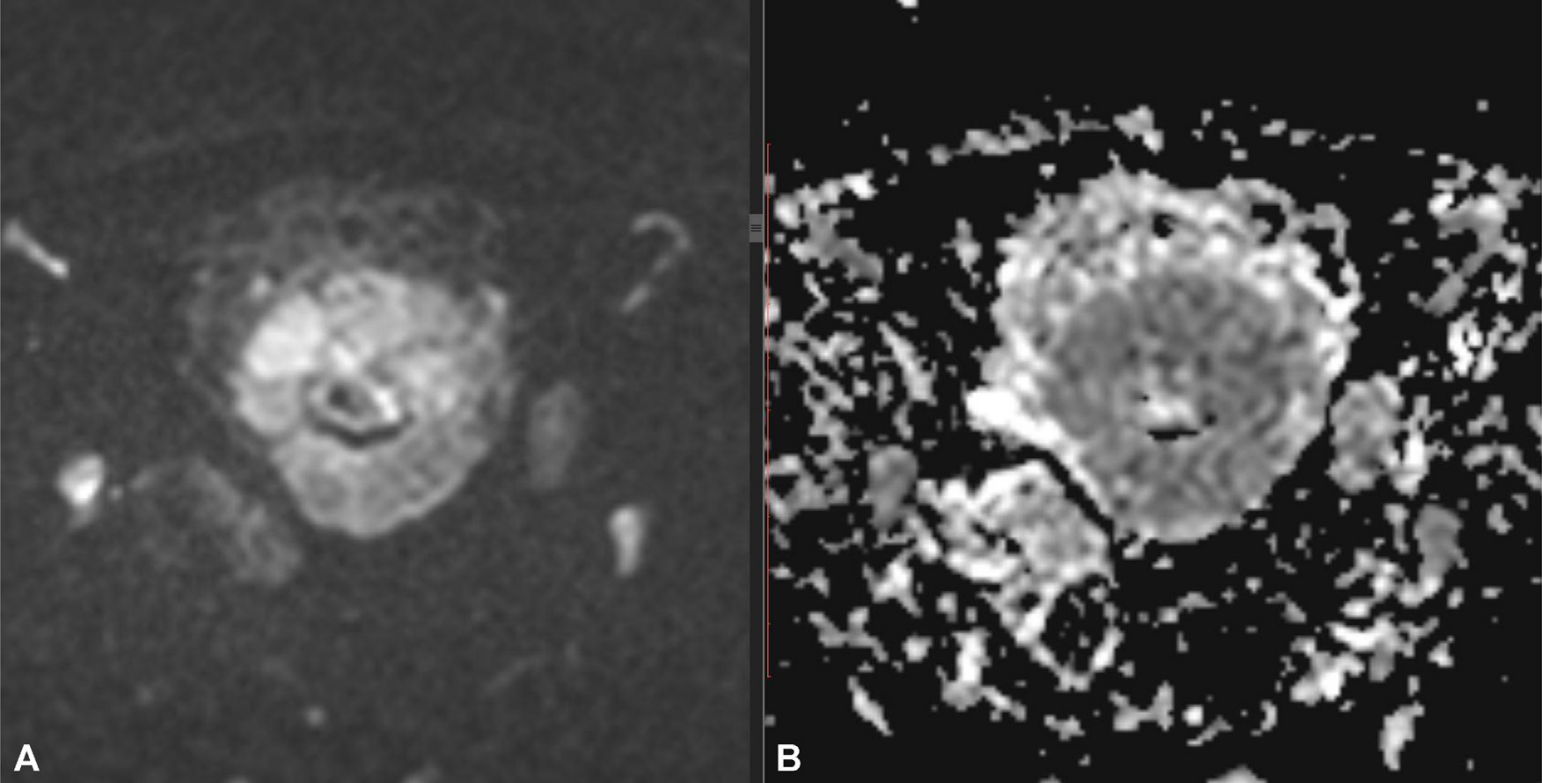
Diffusion weighted images. Infiltrative uterine mass show restricted diffusion seen as hyper intense signals on DWI and hypo intensity on ADC. Associated involved pelvic sidewall lymph nodes also show restricted diffusion.
